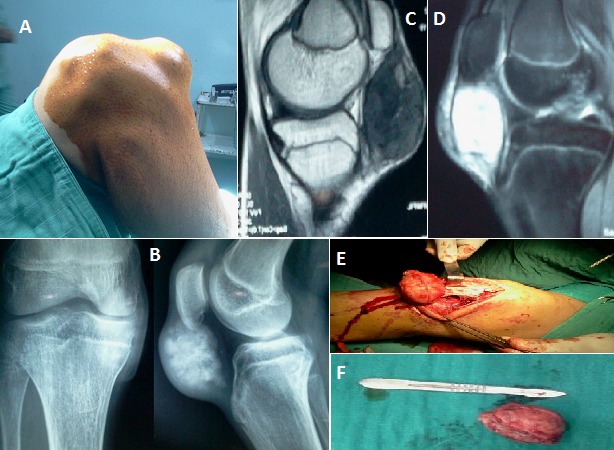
(A): image clinique: masse infra-patellaire du genou droit étendue de la pointe de la rotule à la TTA; (B): radiographie standard du genou droit face + profil: image infra-patellaire bien limitée avec calcifications évoquant la nature cartilagineuse de la tumeur; (C): IRM du genou droit: tumeur ovoïde en hypo-signal en T1; (D): IRM du genou droit: Tumeur ovoïde en hyper-signal en T2; (E): résection marginale de la tumeur facilement décollée sans lésions du tendon rotulien; (F): pièce opératoire: étude anatomopathologique
